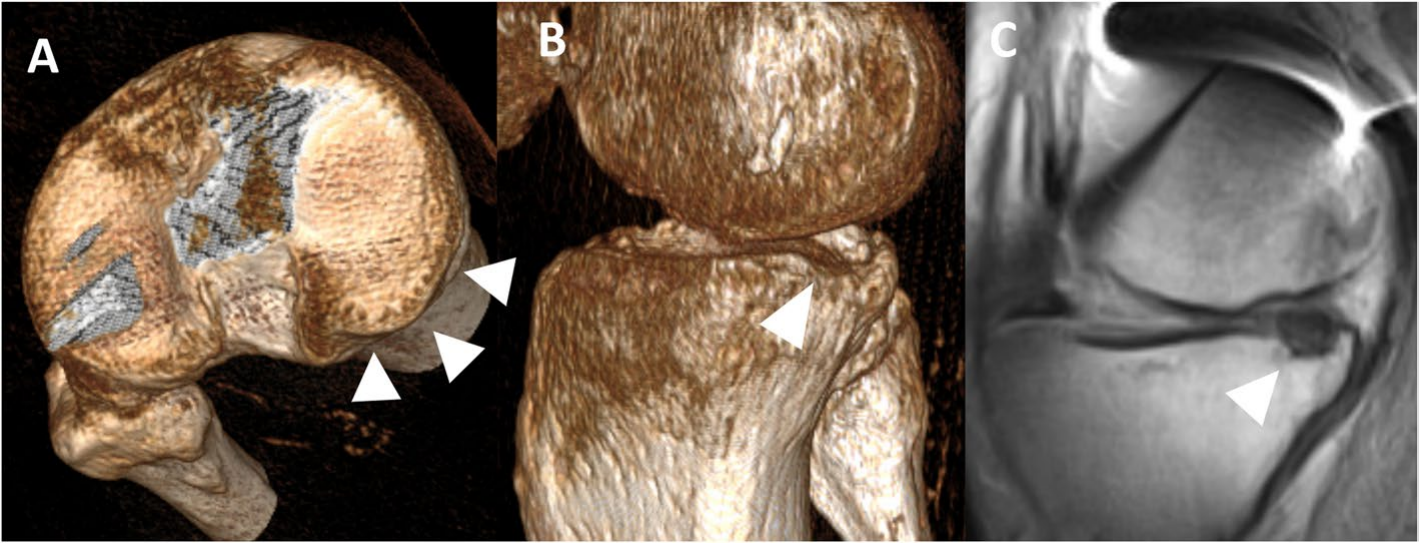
Depression fracture of posterior part of medial tibial condyle at initial presentation to authors’ clinic could be observed on CT (Fig. 3A, Fig. 3B) and MRI (Fig. 3C)
